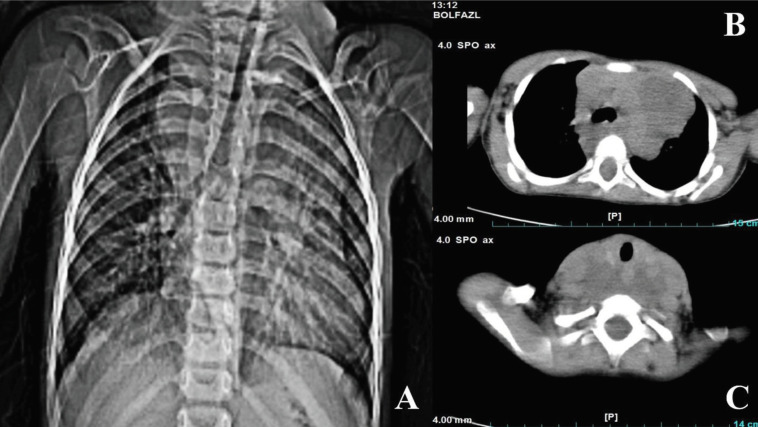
A large mediastinal mass in chest X-ray (2-A) and a heterogenous mass in chest CT scan of thoraces (2-B) with extension to neck (2-C)
